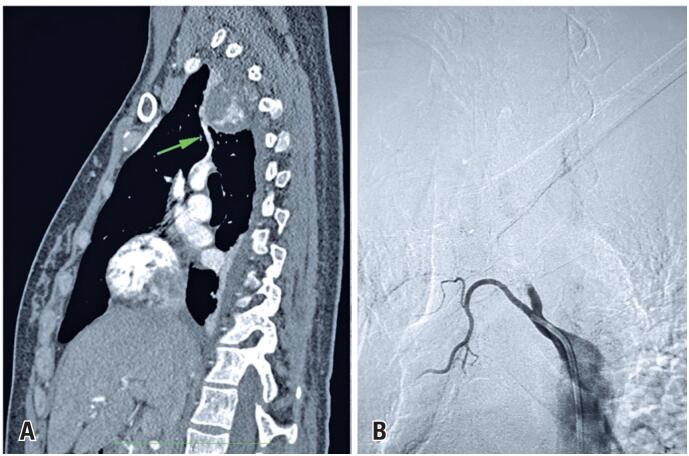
Endovascular embolization. The green arrow on the computed tomography scan demonstrates the vessel nourishing the neoplastic mass (A). After embolization, the flow to the tumor mass is interrupted (B)
